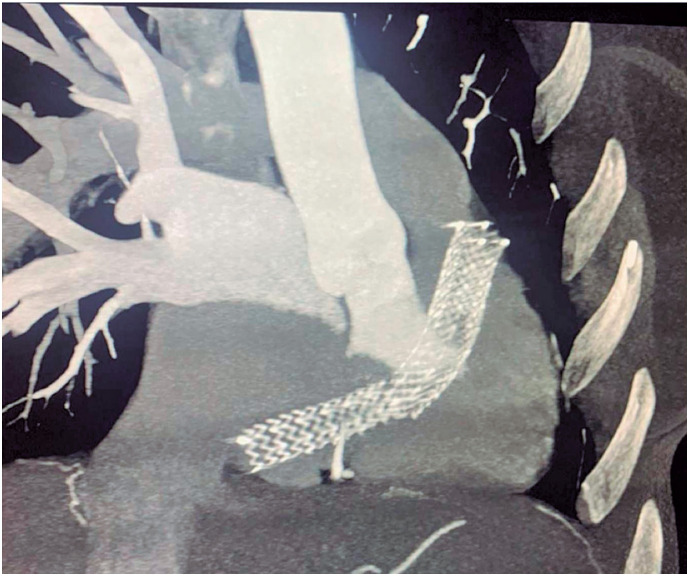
Chest CT angiogram showing the vascular stent in the right heart chambers
